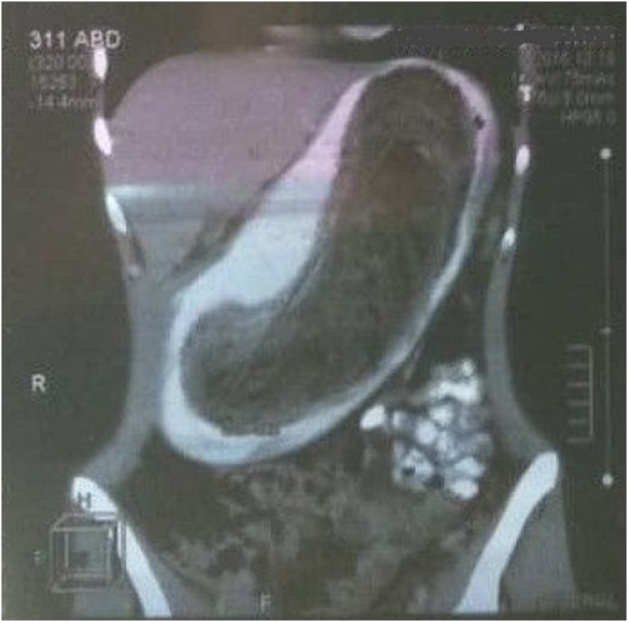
CT scan showing obstructer mass in the stomach.
